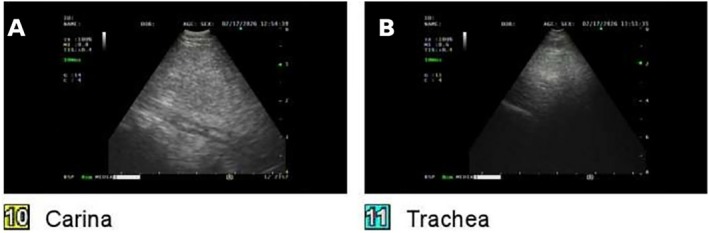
Intraoperative EBUS ultrasound images during bronchoscopic sampling (17 February 2026). (A) EBUS view at the level of the carina demonstrating the mediastinal mass as a homogeneous echogenic structure immediately adjacent to the airway wall, used to guide transbronchial tract creation and cryoprobe positioning. (B) EBUS view from the trachea confirming the superior extent of the mass under real‐time ultrasound visualization.
